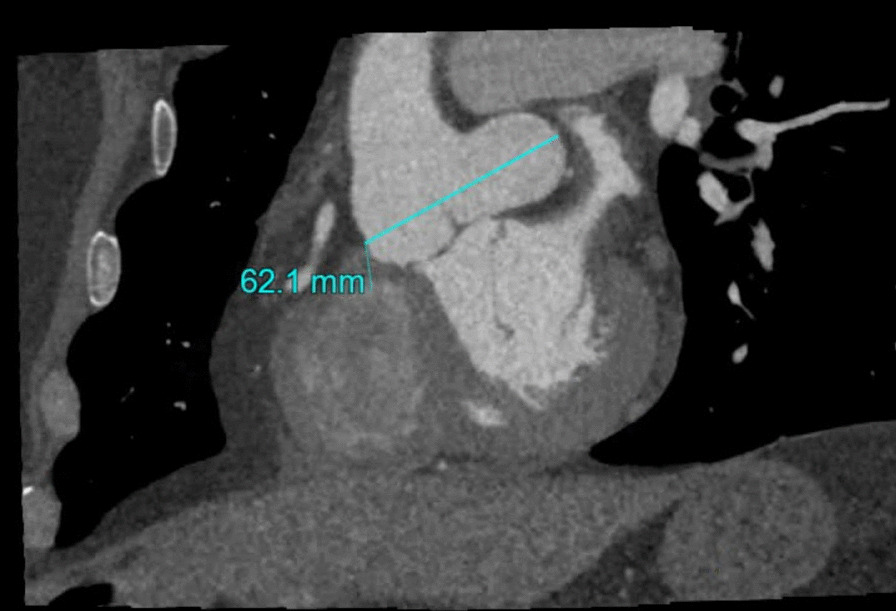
Cardiac CT showing the dilated sinus and the left SOVA
